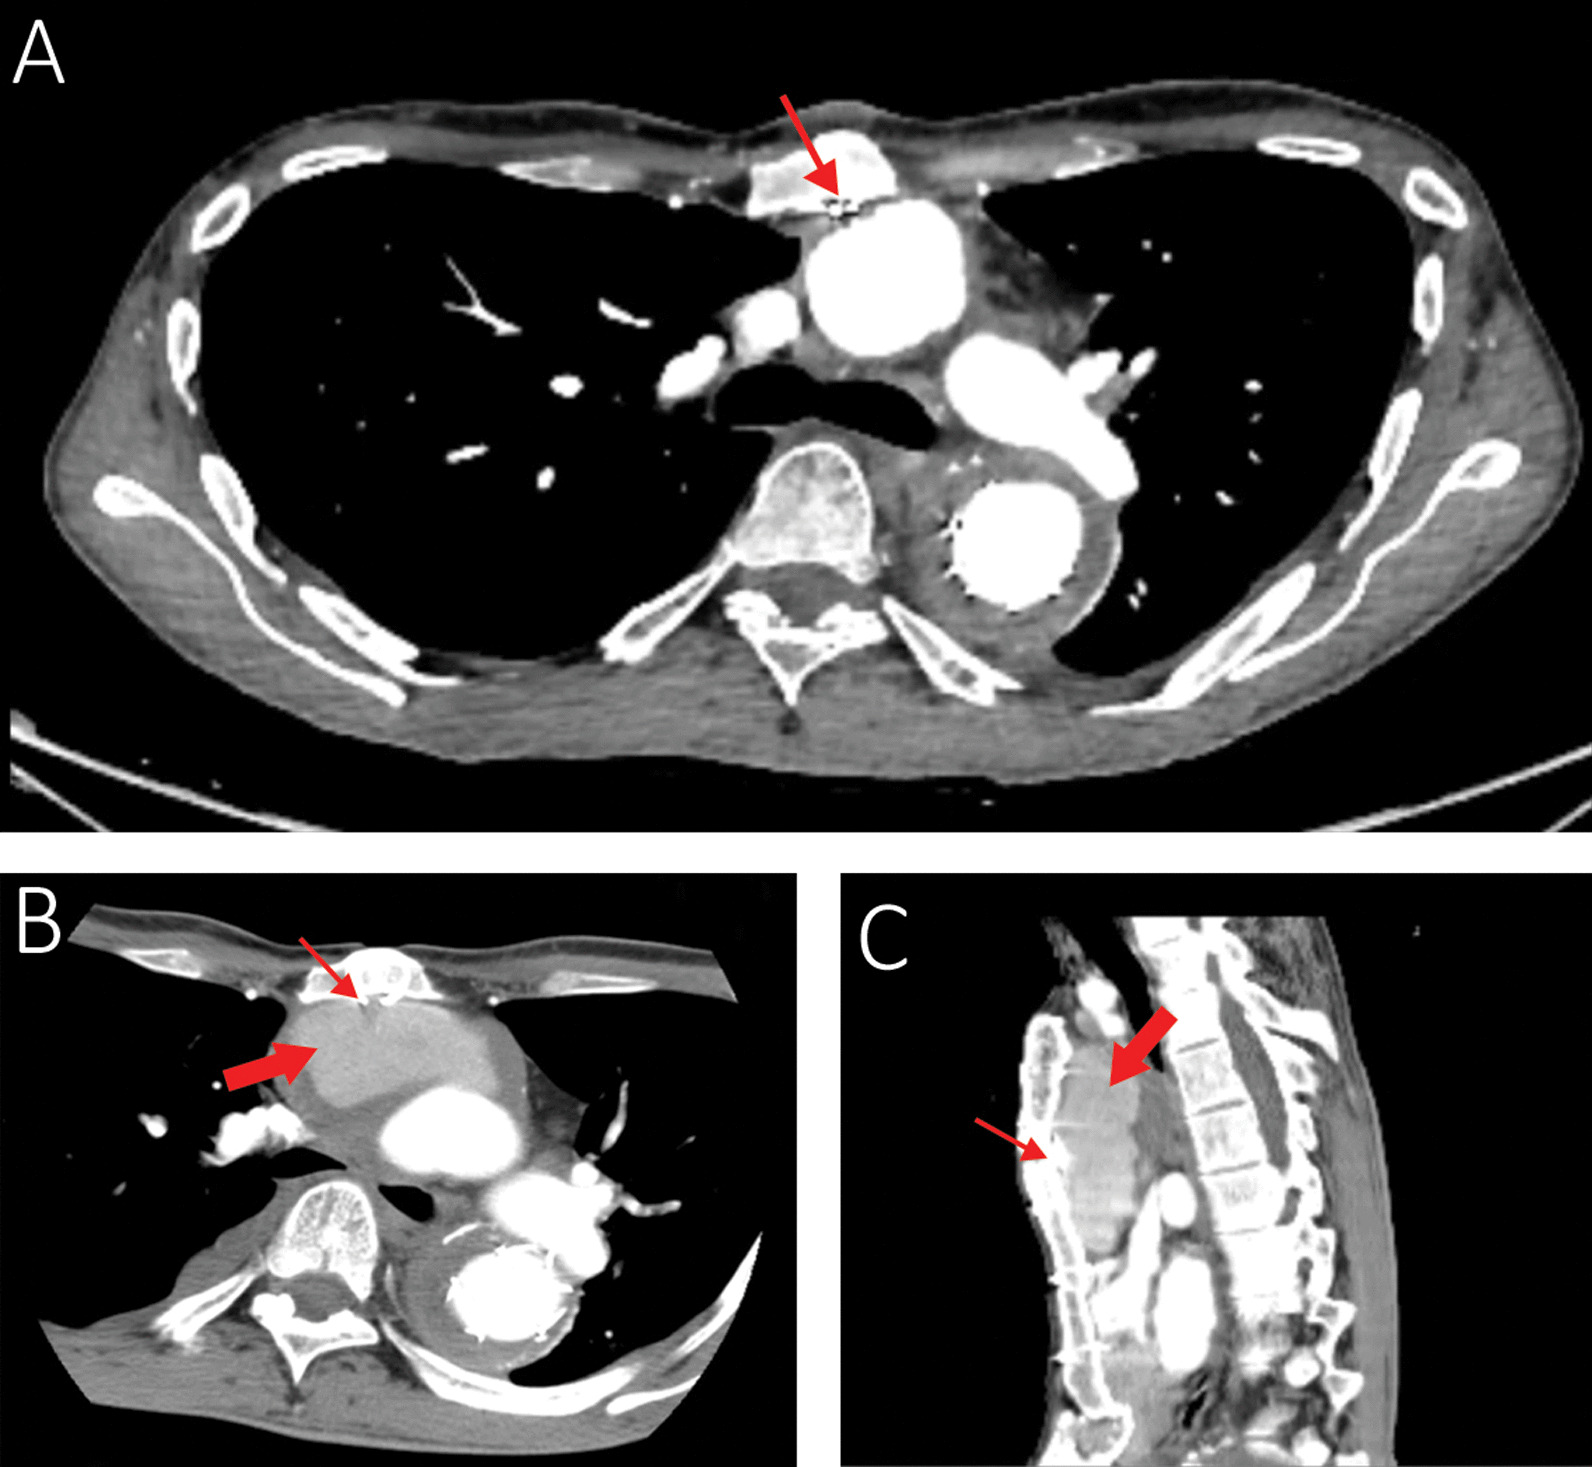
A The CTA performed six months before presenting the hemoptysis. Note the proximity of the sternal wire and the graft and the absence of any space between the sternum and the aortic graft. B and C The recent CTA (axial and lateral views): the thin arrow shows the fractured wire, and the thick arrow shows the pseudoaneurysm. Note the large space filled with a hematoma between the graft and the posterior border of the sternum
